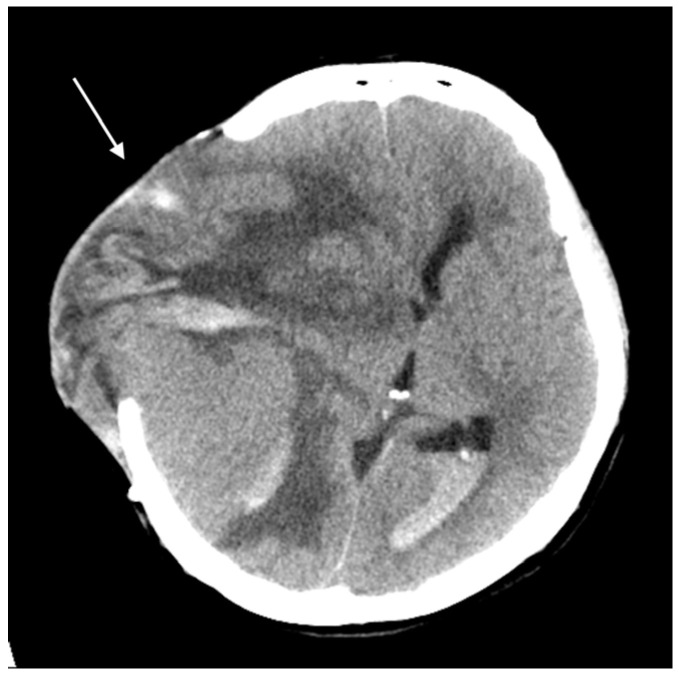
Brain CT without contrast showed a huge recurrent tumor with hemorrhage and cerebral edema (arrow).
